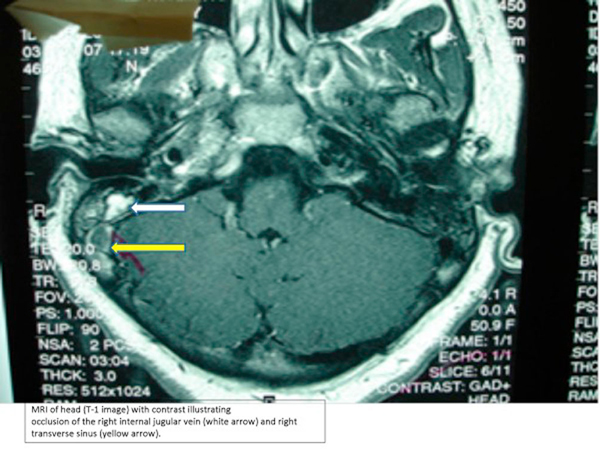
Magnetic resonance imaging of the head: Right jugular venous thrombosis (white arrow) and right transverse sinus thrombosis (yellow arrow).
